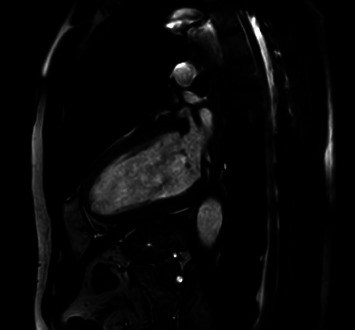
Cardiac MRI imaging at initial diagnosis after cardiovascular bypass surgery: infarct scar (over 75% transmural) on the left ventricular posterior wall and a normally dimensioned left ventricle with significantly reduced left ventricular function.
